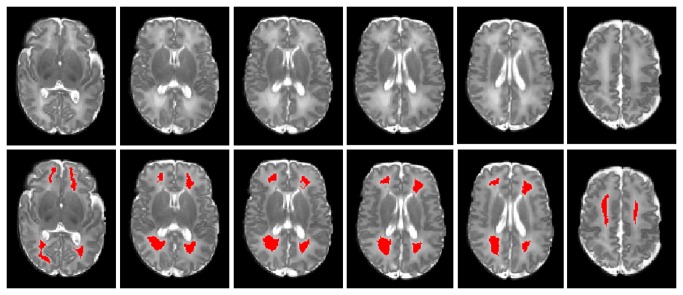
Automated WMSA detection at six different axial levels. Top row: T2-weighted images; bottom row: detected WMSA marked in red. The automated detections closely approximated the visually apparent signal abnormalities.
